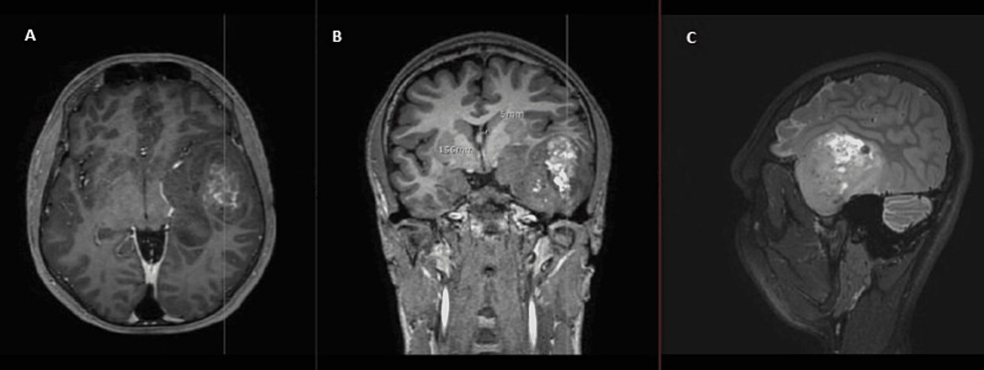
Cranial MRI showing the first lesion in the left temporal area (T1 sequences with contrast, before surgery) (A) Axial view; (B) coronal view; (C) sagittal view The distance from the midline to the lateral border of the tumor is 156 mm; the distance from the midline to the medial border is 5 mm, in the coronal section. Image courtesy: Al Zahraa Hospital University Medical Center (ZHUMC) Radiology Department
